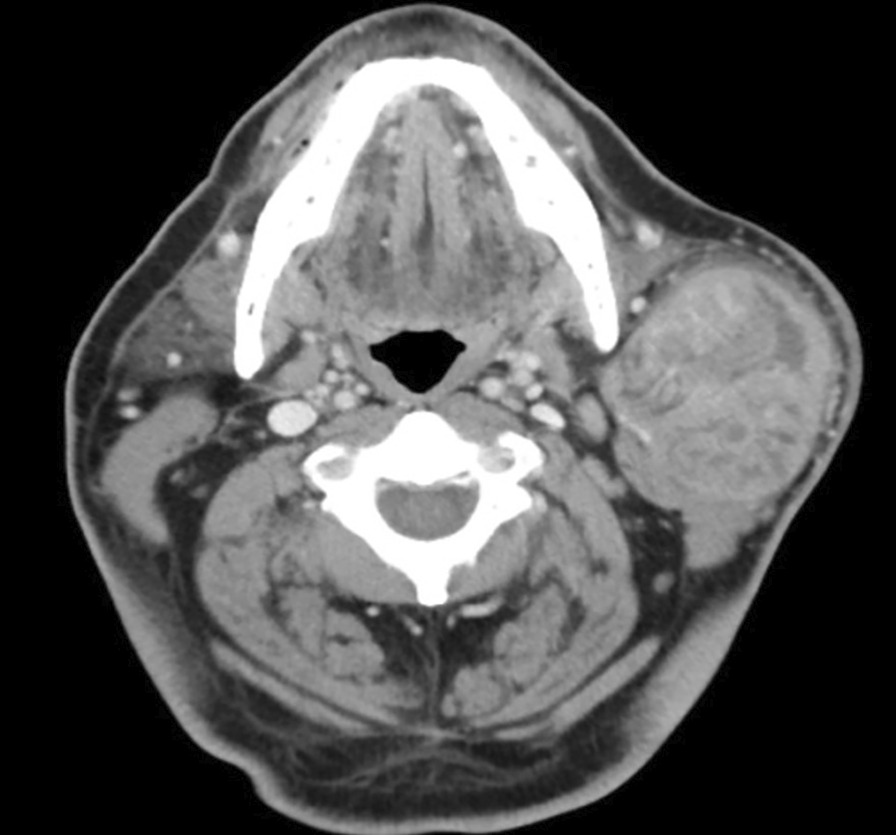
Computed tomography scan, coronal view: well-defined nodule with heterogeneous enhancement and multiple cystic spaces in the left parotid gland
